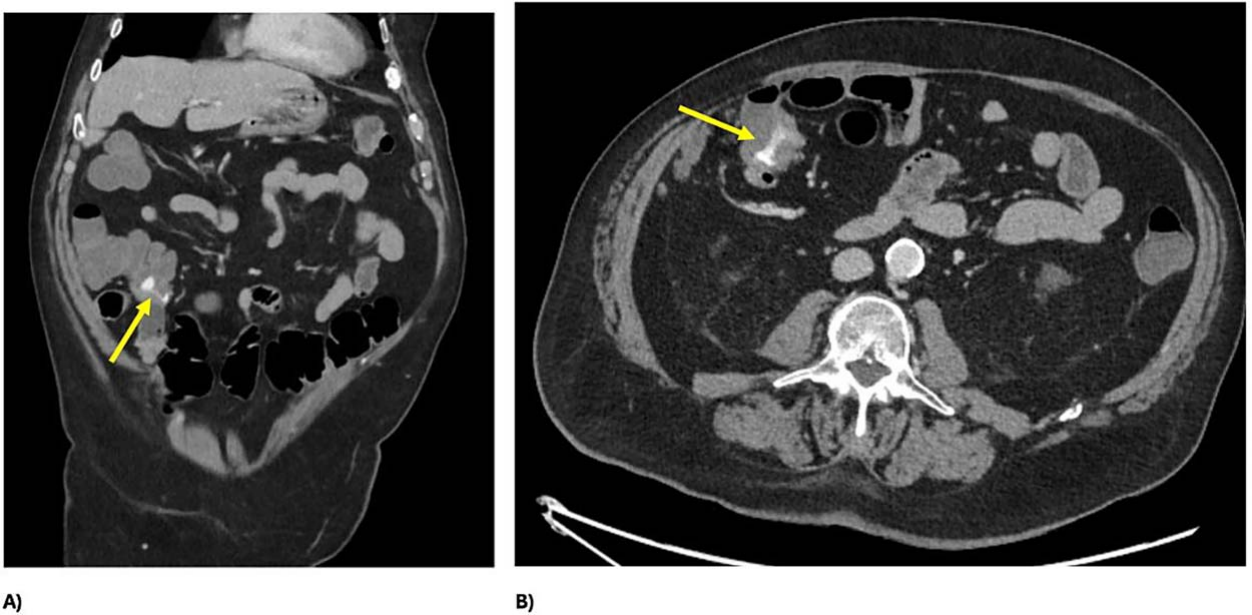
CT demonstrating active bleeding pre-embolization, with pooling of contrast in the caecum and appendiceal base; (A) axial view and (B) coronal view.
